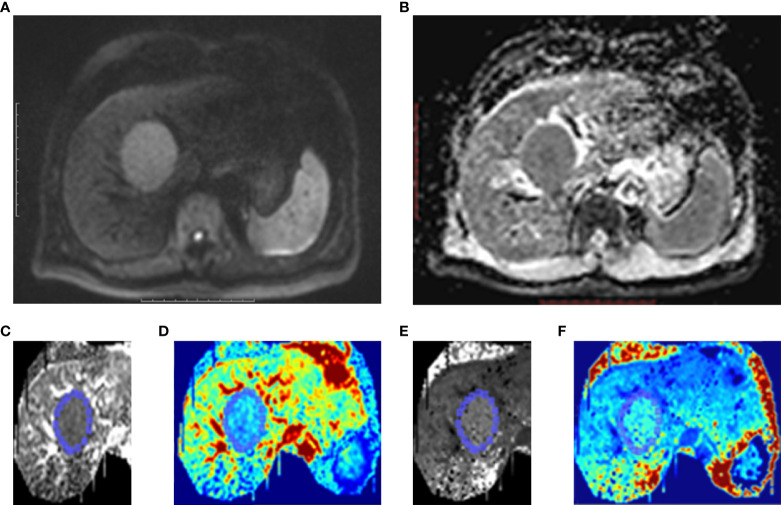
One case of HCC patients without MVI. Male, age 39 years, hepatitis B (+). (A) A lesion located at left lobe of liver showed high signal intensity on Diffusion-weighted imaging (DWI). (B) On ADC map, the lesion showed as restricted diffusion. Kurtosis map (C) and corresponding pseudo-color kurtosis images (D) showed lower signal intensity compared with that of liver parenchyma. Diffusion map (E) and corresponding pseudo-color diffusion images (F) showed iso-intensity compared with that of liver parenchyma.
